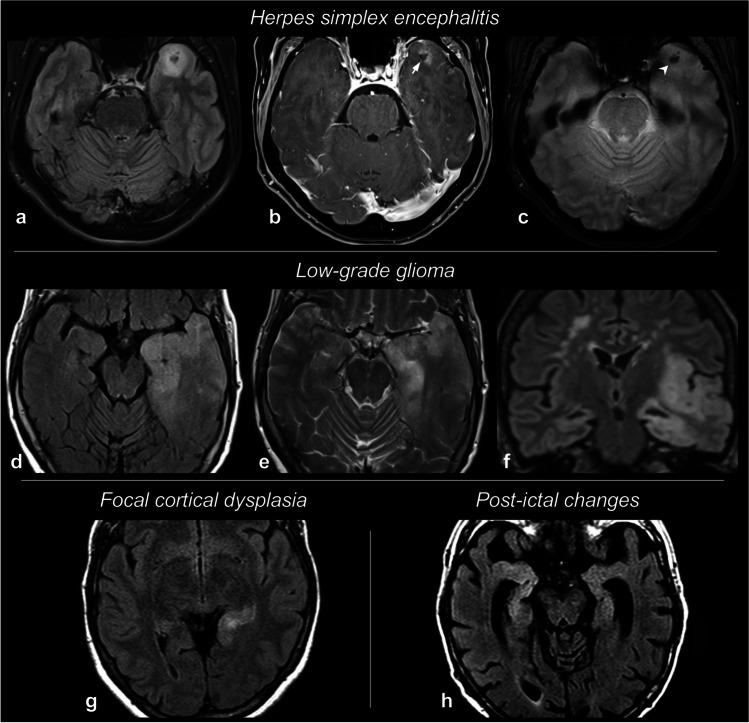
Differential diagnoses of autoimmune encephalitis. Some examples of conditions mimicking limbic autoimmune encephalitis are collected here. In a typical case of herpes simplex encephalitis (HSE) (a–c), unilateral T2-weighted FLAIR hyperintensity of the left anterior temporal areas (a) is associated with post-contrast T1 focal enhancement, with a central non-enhancing and hypointense spot (b, arrow), corresponding to hemorrhagic foci on T2*-weighted gradient-echo images (c, arrowhead). In a patient with low-grade glioma (LGG) (d–f), T2-weighted FLAIR (d, f) and T2-weighted TSE (e) exhibit a marked swelling and hyperintensity of the left anterior temporal lobe and insula, with a widespread thickening of the cortex and mass effect on the sulci. An example of focal cortical dysplasia (FCD) located in the tail of the left hippocampus is shown on T2-weighted FLAIR images (g). Finally, T2-weighted FLAIR images (h) obtained after an epileptic seizure demonstrate a MTL signal hyperintensity due to post-ictal changes
